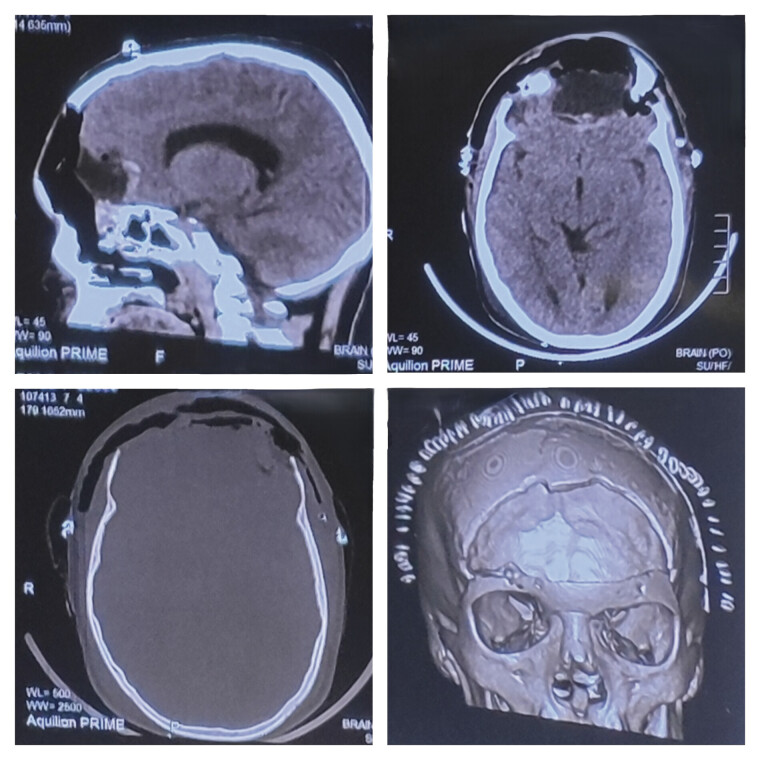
Postoperative computed tomography (CT) scan. Clockwise—sagittal, axial, three-dimensional reconstruction, and bone window axial images showing near-complete excision of the lesion with a bony defect in bilateral frontal region post bifrontal craniotomy.
